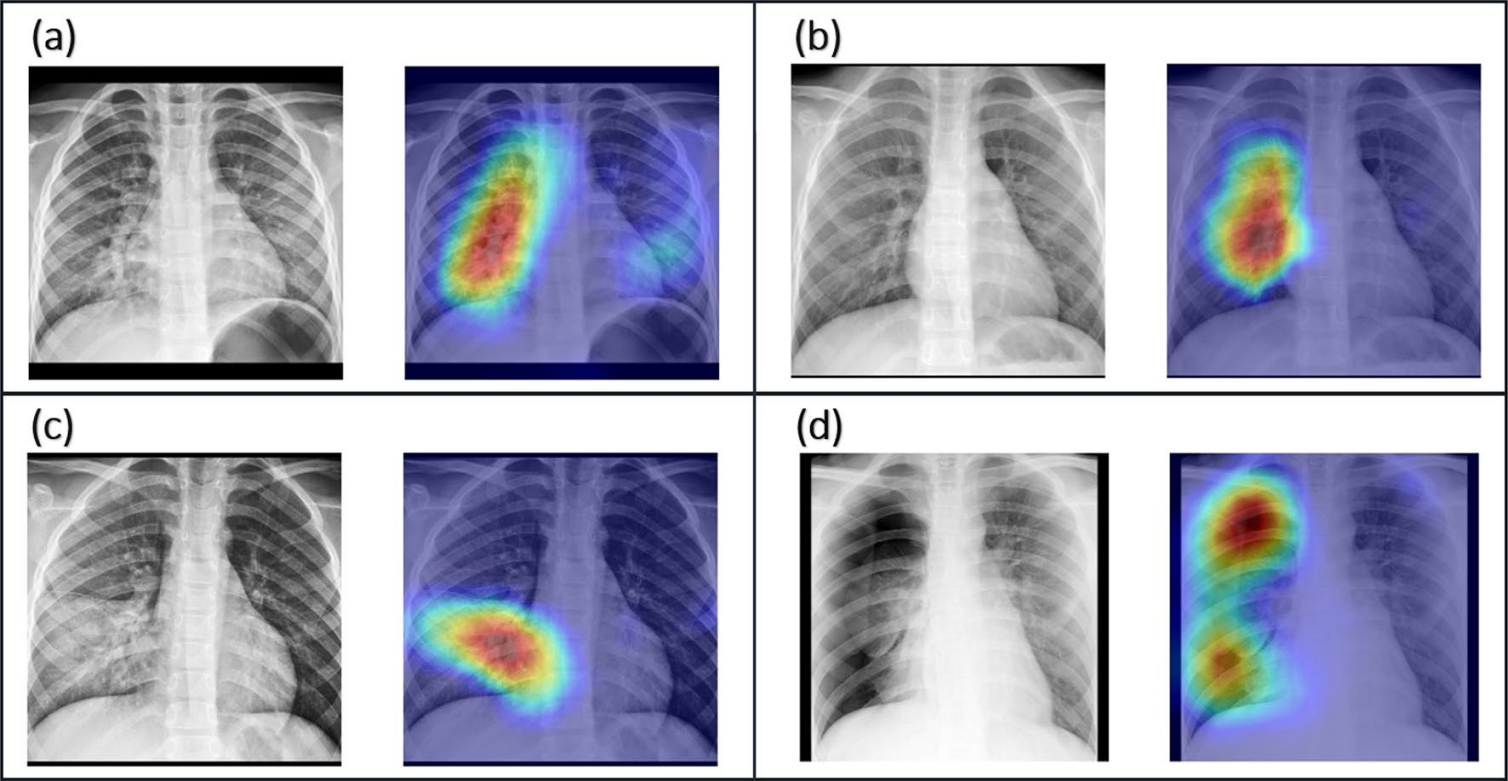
Image pairs of radiographs and the corresponding Grad-CAM of the test set: (a) bronchopneumonia, (b) bronchiolitis, (c) lobar pneumonia, (d) pneumothorax.
